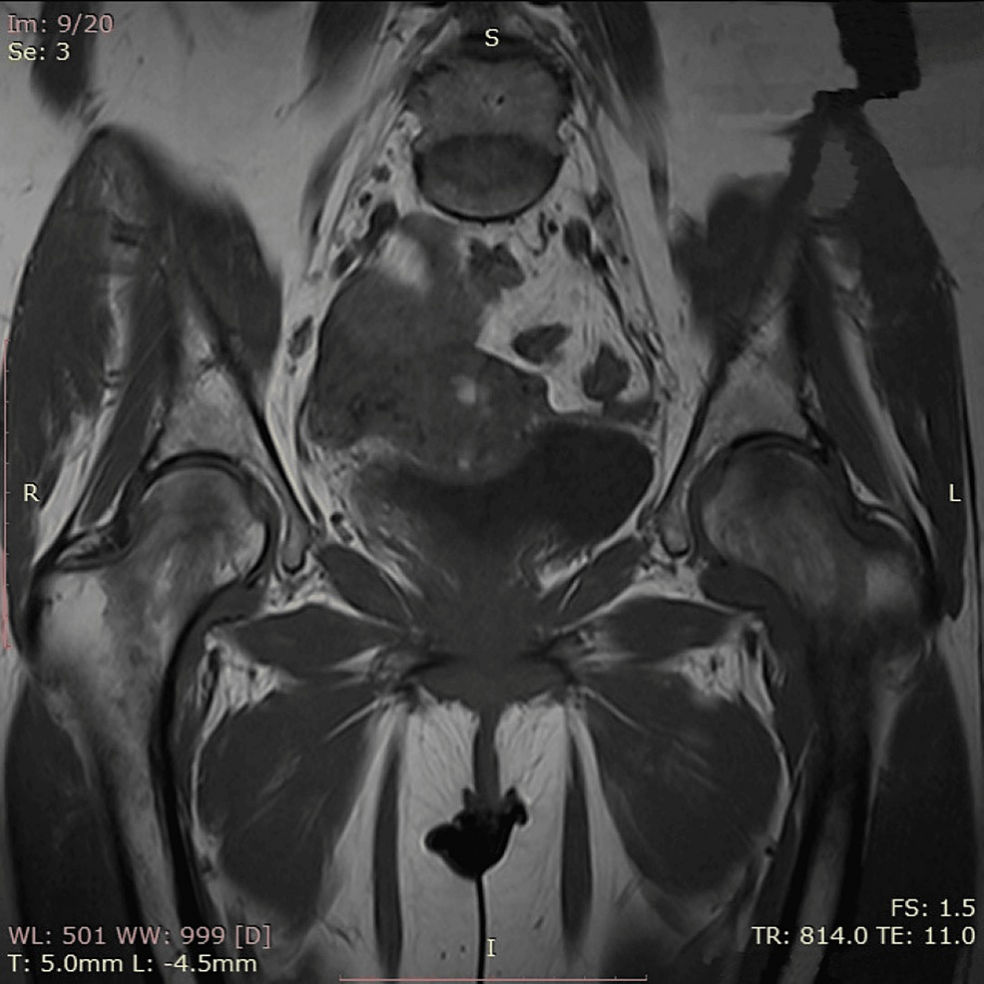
Magnetic resonance imaging of bilateral transient pregnancy-related osteoporosis of the hip. After the administration of intravenous contrast material, no abnormal enhancement of the bone marrow in either femur was detected. This absence of abnormal enhancement rules out the presence of tumors (primary or metastatic), infections (osteomyelitis), or other inflammatory conditions.
